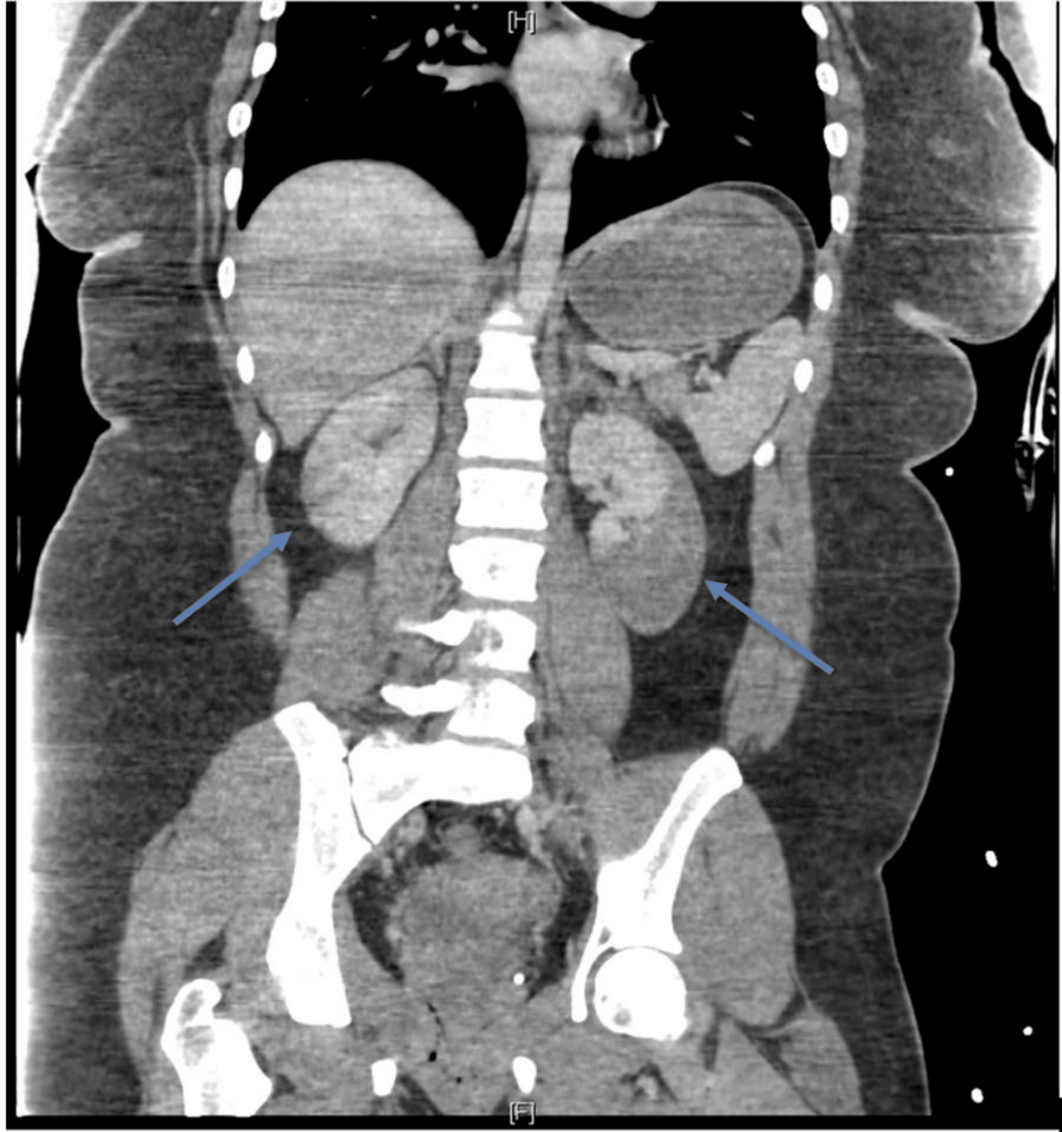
Contrast-enhanced coronal-view CT scan showing bilateral renal infarcts The blue arrows point to the infarcted right and left kidneys
